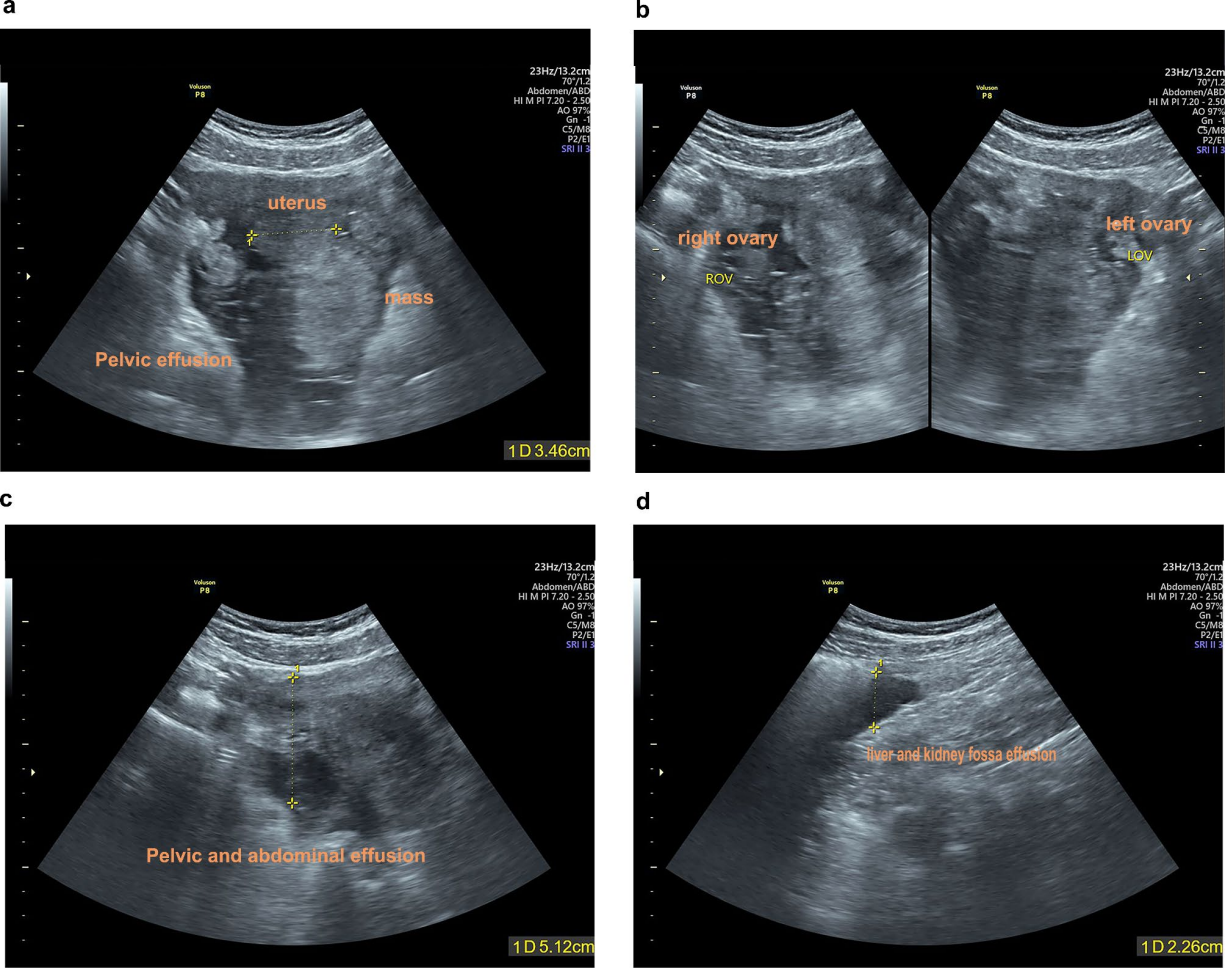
Ultrasound Imaging before operation. (a) An uneven slightly higher echo photophore is found at the back of the uterus and is surrounded by a large volume of fluid. (b) Normal morphology of the left and right ovary. (c) Pelvic and abdominal effusion. (d) Liver and kidney fossa effusion
